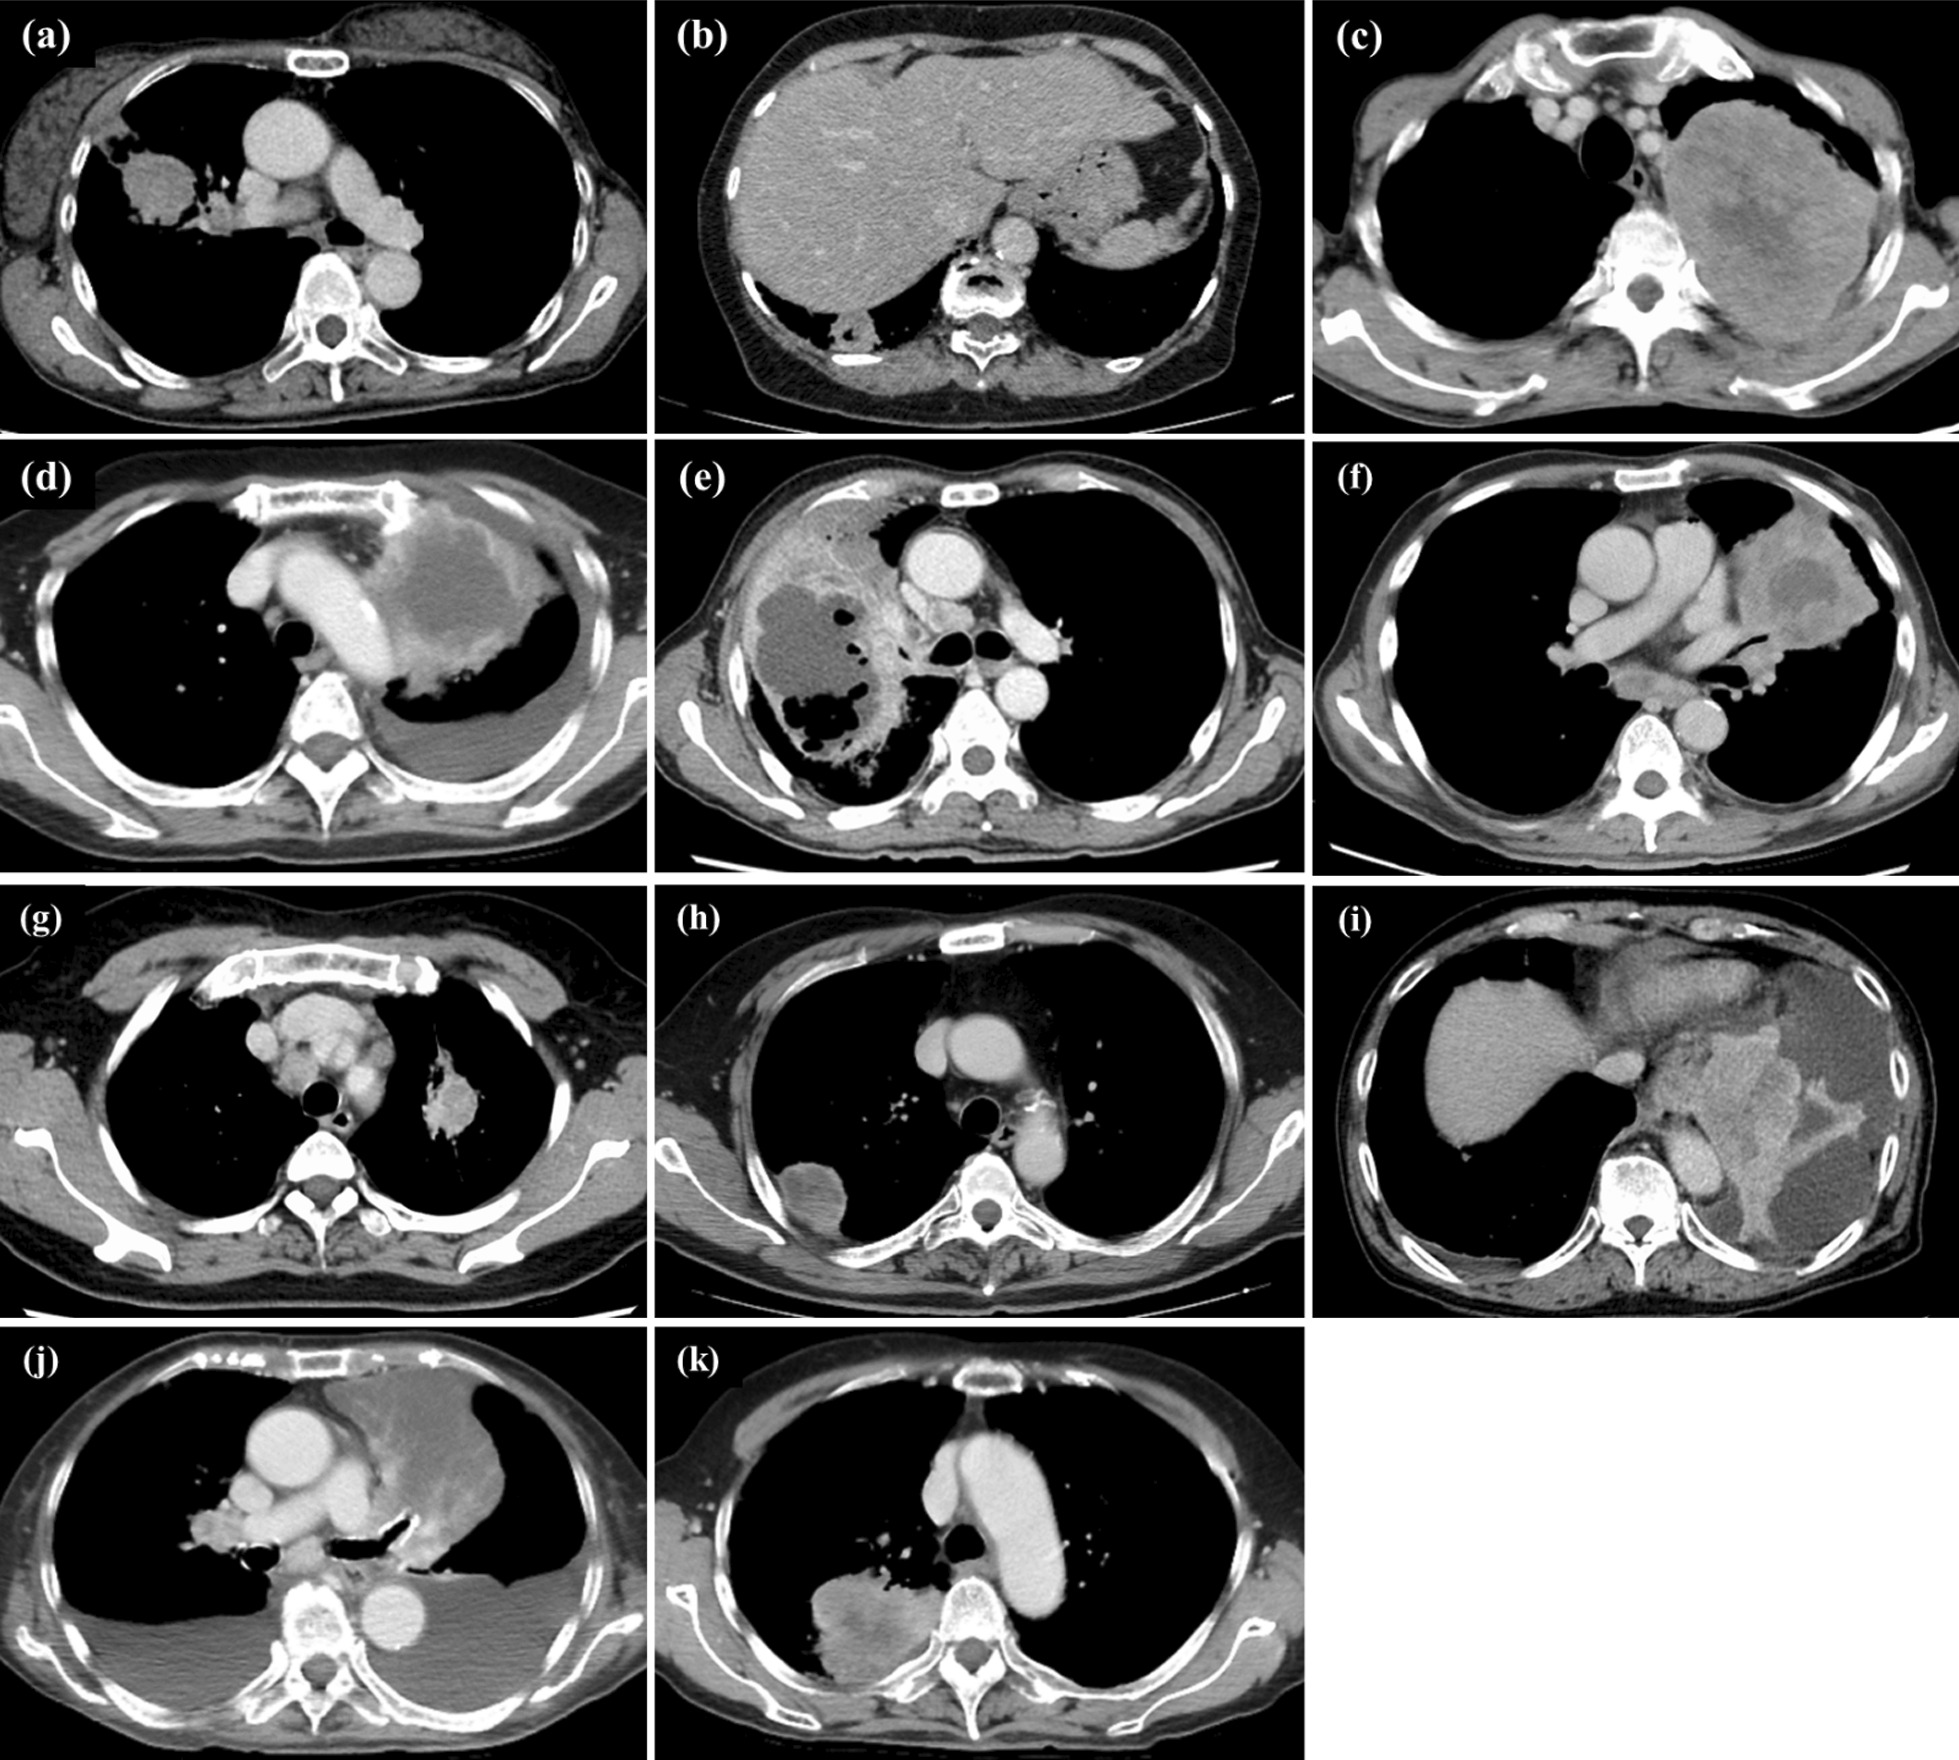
CT images of stage III/IV NSCLC with METex14 skipping. a–e are CT images of patients with stage III NSCLC, and f–k are those of patients with stage IV NSCLC. a does not show invasion into surrounding tissue nor internal low-density areas. b–e and h–k show both invasion into the surrounding tissue and internal low-density areas. f and g show the internal low-density areas
